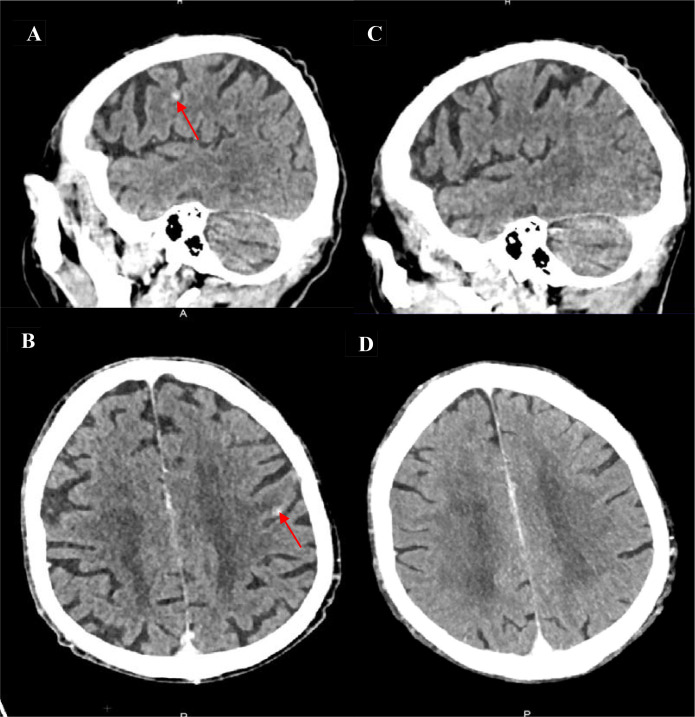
Brain involvement and response to trastuzumab–deruxtecan in Case 1. (A, B) Baseline brain CT showing a left parietal metastasis with contrast enhancement and perilesional edema; the red arrows highlight the metastatic lesion on both images (A Sagittal; B Axial). (C, D) Follow-up CT after the initiation of trastuzumab–deruxtecan demonstrating complete disappearance of the lesion previously indicated by red arrows (C Sagittal; D Axial).
